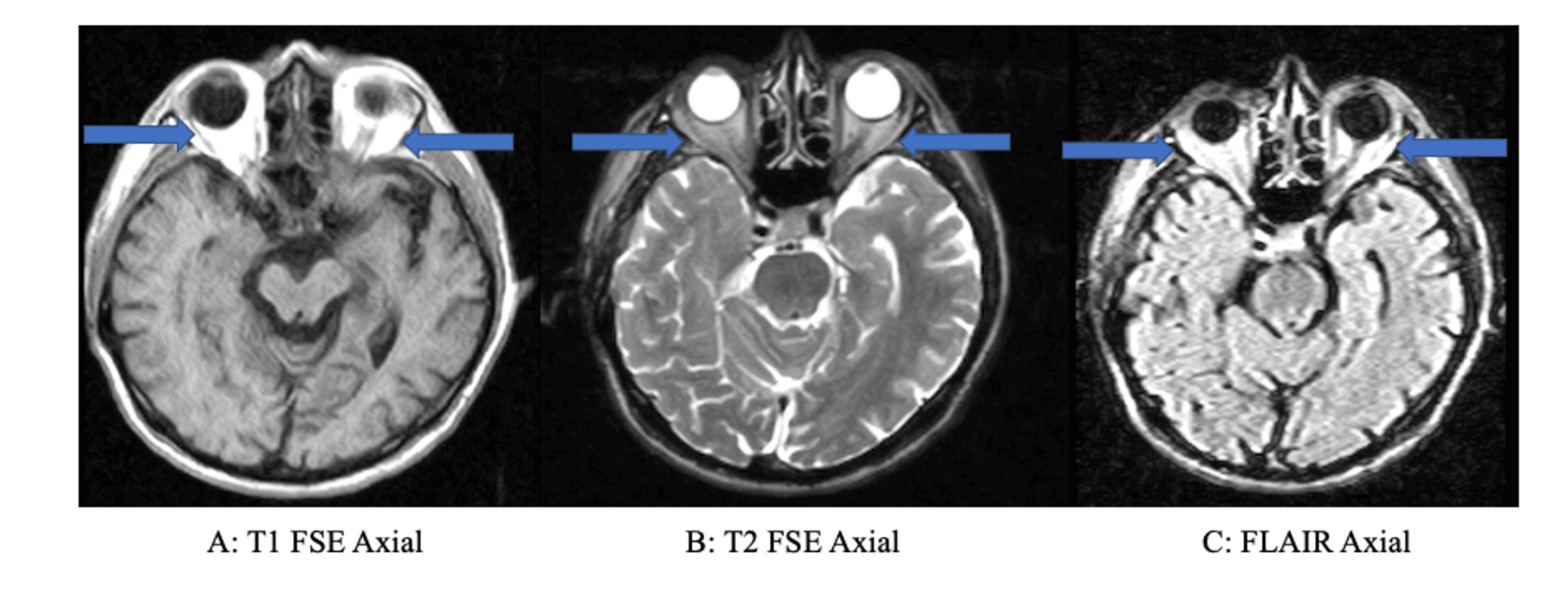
MRI of orbitals MRI: Magnetic resonance imaging; FSE: fast spin-echo; FLAIR: fluid-attenuated inversion recovery This figure demonstrates the orbits, depicted by the blue arrows. The orbits were noted to be grossly unremarkable; however, the radiologist noted that the MRI study was limited due to the signal-to-noise ratio. A depicts the brain MRI and orbits in T1 FSE axial view, B does so in T2 FSE axial view, and C does so with FLAIR axial view
